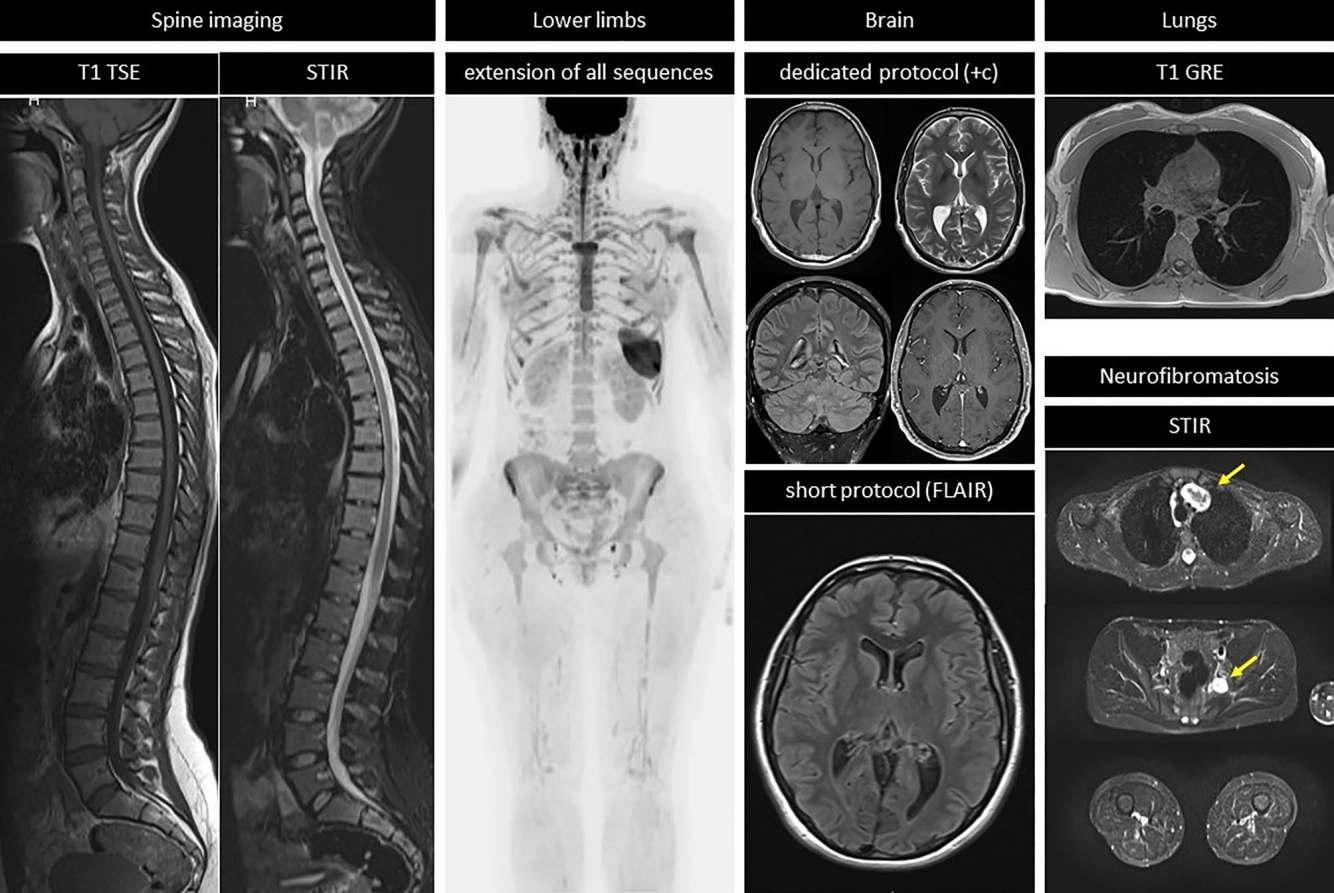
Extensions to the core protocol. This picture summarizes possible complements to the core protocol. When assessment of the spine is required, sagittal short tau inversion recovery (STIR) is used. In subjects with a high risk of vertebral tumours, a sagittal T1 weighted turbo spin echo (TSE) sequence can be additionally performed. When the WB-MRI protocol includes the lower limbs, such as in subjects with Li Fraumeni syndrome, all sequences in the core protocol are extended to the feet. When there is an increased risk of central nervous system (CNS) tumours, a dedicated brain sub-protocol is performed, with multiple sequences and with contrast administration (+ c). In subjects with a low risk of CNS tumours, the assessment of the brain can be improved with a short brain protocol, including fluid attenuated inversion recovery (FLAIR) sequences. Single breath-hold T1 weighted gradient recalled echo (GRE) sequences can be performed for the assessment of the lungs. In subjects with neurofibromatosis, the STIR sequence should be performed in either the axial or the coronal plane, covering from neck to feet, for facilitating the detection of peripheral nerve sheath tumours (arrows)
